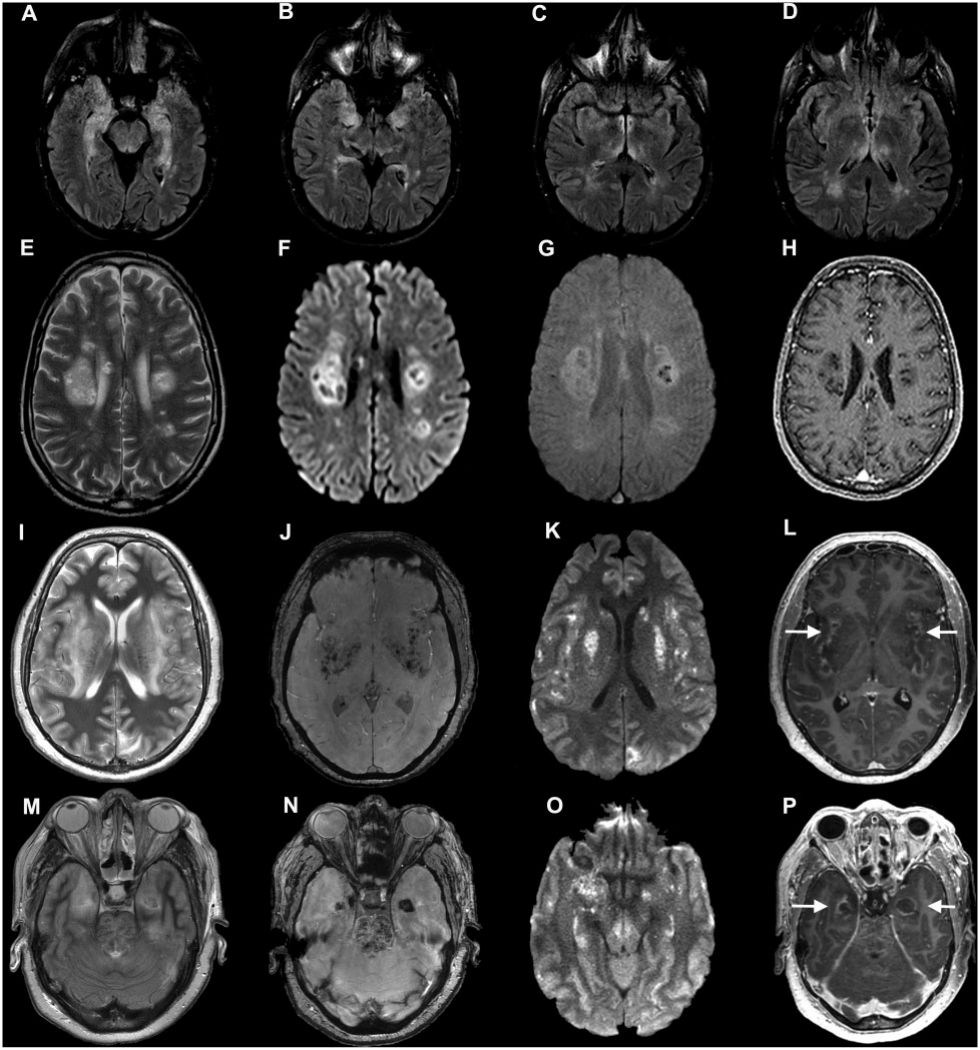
Imaging from Patients 12, 13 and 15 (COVID-19 autoimmune and haemorrhagic encephalitis). Axial MRI from three individuals with para-/post-infectious central syndromes. (A–D) Patient 12: axial fluid-attenuated inversion recovery (FLAIR) images show bilateral hyperintensity in the mesial temporal lobes (A and B), hypothalamus (C) temporal lobes and thalamus (D). (E–H) Patient 13: axial T_2_-weighted (E), diffusion weighted imaging (DWI) (F), susceptibility weighted imaging (SWI) (G) and post-contrast T_1_-weighted (H) images show multifocal clusters of lesions involving the deep white matter of both cerebral hemispheres, intralesional cyst-like areas of varied sizes, and some peripheral rims of restricted diffusion (F), some haemorrhagic changes (G), and T_1_ hypointense ‘black holes’ without contrast enhancement (H). (I–P) Patient 15: axial images at the level of the insula and basal ganglia (I–L) and at the level of the temporal lobes and upper pons (M–P). T_2_-weighted images (I and M), SWI images (J and N), DWI images (K and O) and contrast-enhanced images (L and P). There are extensive confluent areas of T_2_ hyperintensity (I and M), with haemorrhagic change on SWI imaging (J and N), restricted diffusion on DWI images (K and O) and peripheral contrast-enhancement (arrows in L and P) in the insular region, basal ganglia and left occipital lobe (I–L) as well as in the medial temporal lobes and upper pons (M–P).
